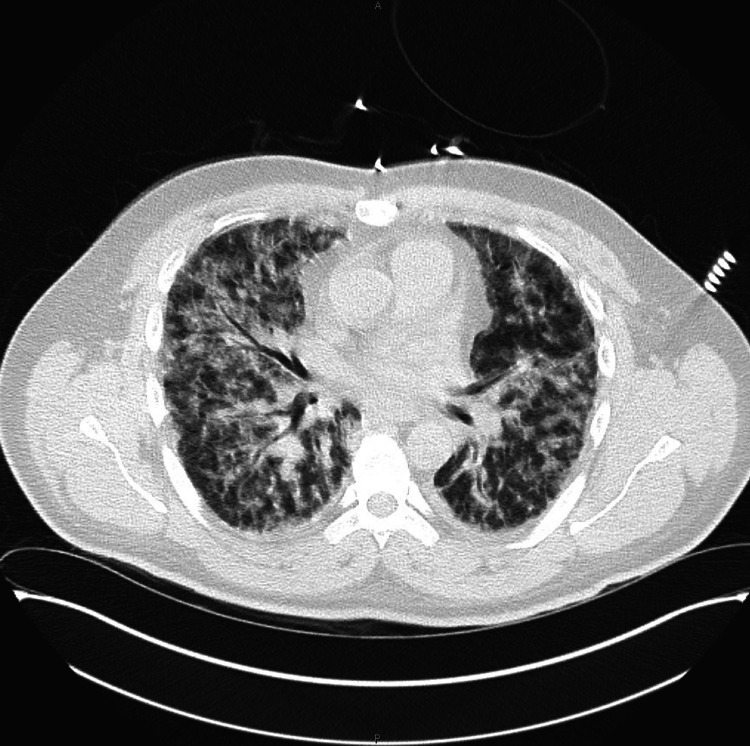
CT chest showing severe progressive diffuse interstitial and airspace lung disease of uncertain etiology The appearance is not typical for community-acquired pneumonia or congestive heart failure/fluid overload.
